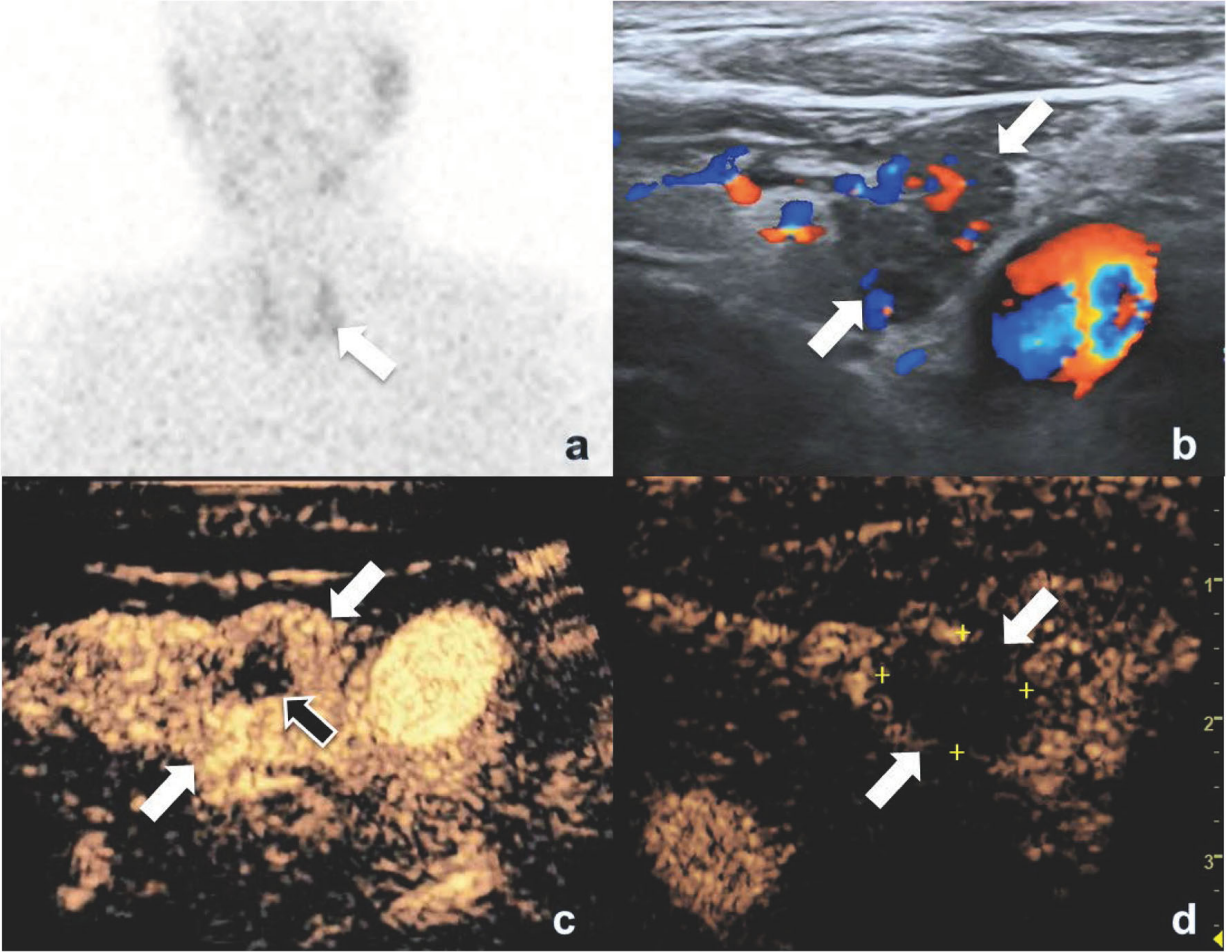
CEUS shows a hyperenhanced residual lesion in a 75-year male patient with pHPT. a Three months after the first MWA, there was radioactive concentration in residual lesion (arrow) on MIBI scan. b Color Doppler showed abundant blood flow signals around the hypoechoic ablation zone (arrows). c There was active area—annular hyperenhancement area—(white arrows) around ablation zone (black arrow) on CEUS. d After additional ablation, non-enhancement (arrows) was shown on CEUS. pHPT, primary hyperparathyroidism; CEUS, contrasted-enhanced ultrasound; MWA, microwave ablation; MIBI, technetium 99 m (^99m^Tc) sestamibi
